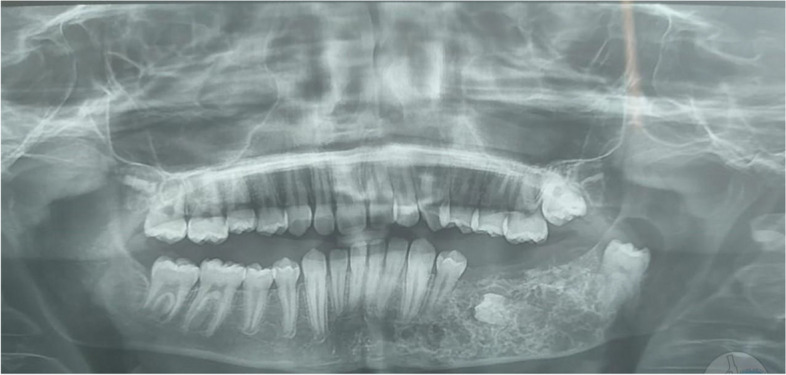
Preoperative panoramic radiograph revealed a well-defined osteolytic multilocular radiolucency at posterior mandible associated with diffuse radiopacities causing displacement of teeth and hindering their eruption
